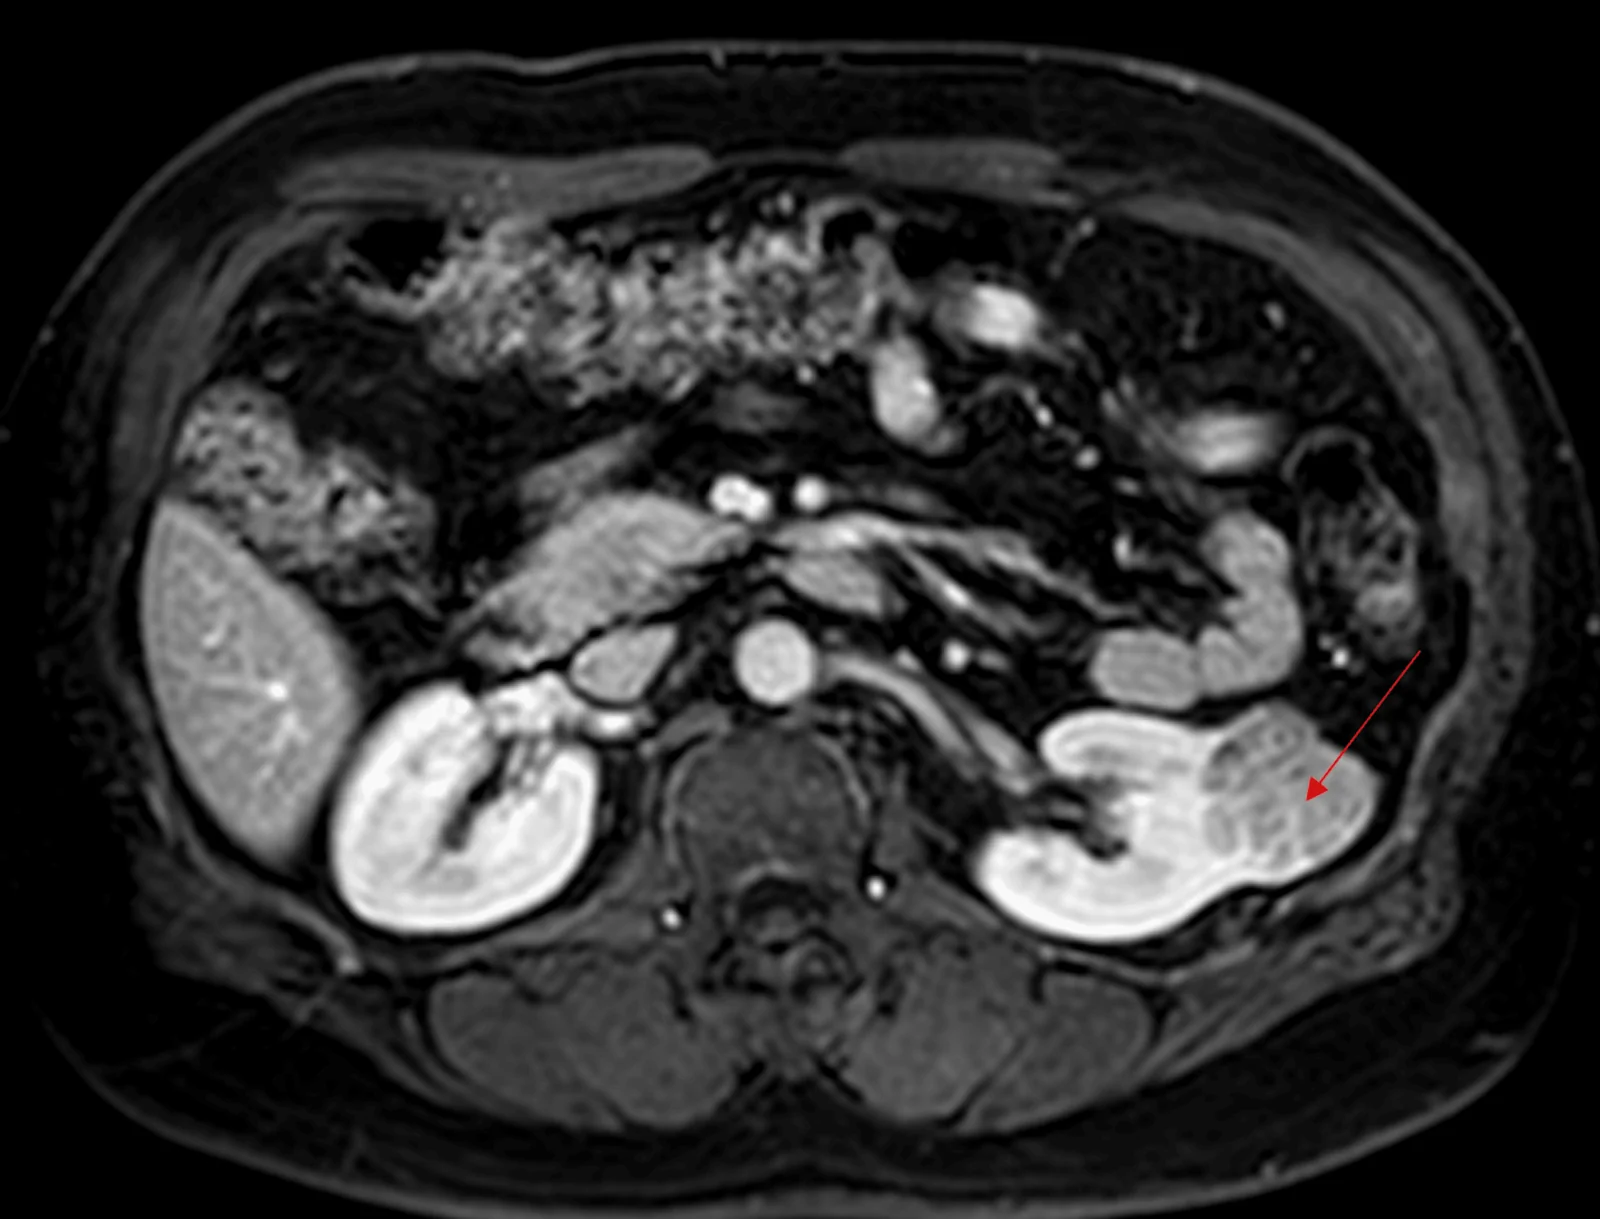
Contrast-enhanced abdominal magnetic resonance imaging, T1-weighted sequence in the portal phase (axial view) An eccentric, heterogeneous renal lesion in the interpolar region of the left kidney with heterogeneous enhancement in the portal phase has a Clear Cell Likelihood Score (ccLS) of 3, indicating intermediate probability for clear cell carcinoma (red arrow).
